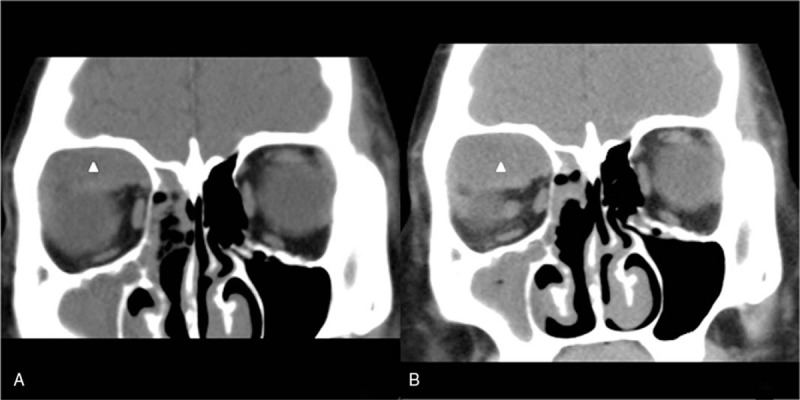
(A) Preoperative coronal computed tomography scan showing a right orbital subperiosteal abscess (white triangle). (B) Postoperative coronal computed tomography scan showing that the right orbital abscess (white triangle) is larger than that preoperatively.
